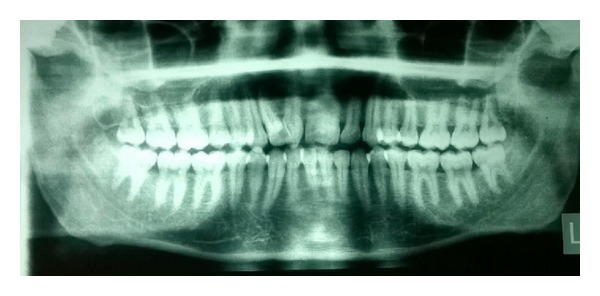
Orthopantomograph showing geminated maxillary central incisors with split roots.
